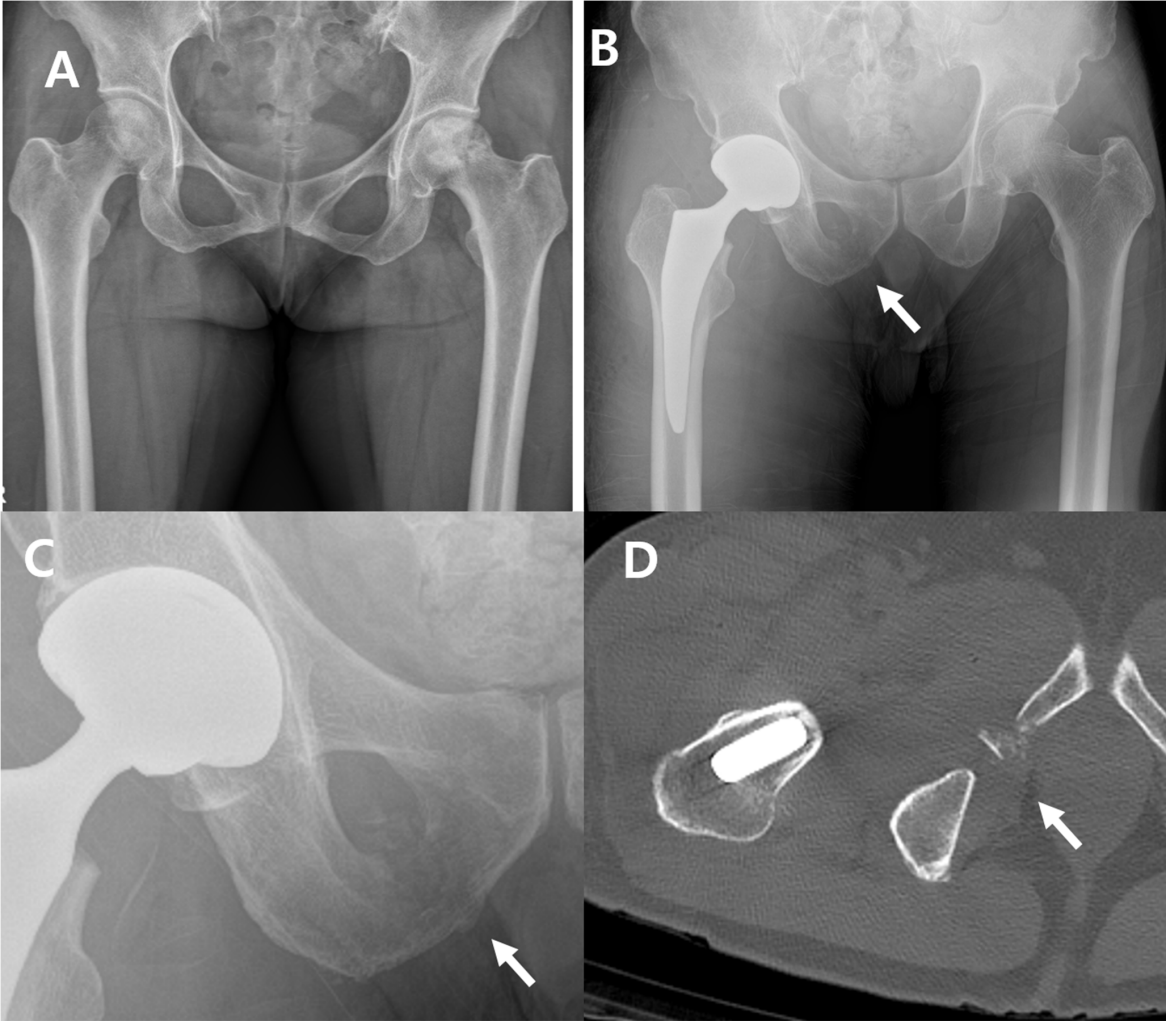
Radiographs showing an inferior pubic ramus fracture during total hip arthroplasty. (A) Preoperative anteroposterior view. (B) Fragmented fractures (arrow) on the right inferior pubic ramus are visible on the postoperative hip anteroposterior radiograph. (C) Magnified view. (D) The postoperative hip computed tomography axial view reveals comminuted fractures on the right inferior pubic ramus
